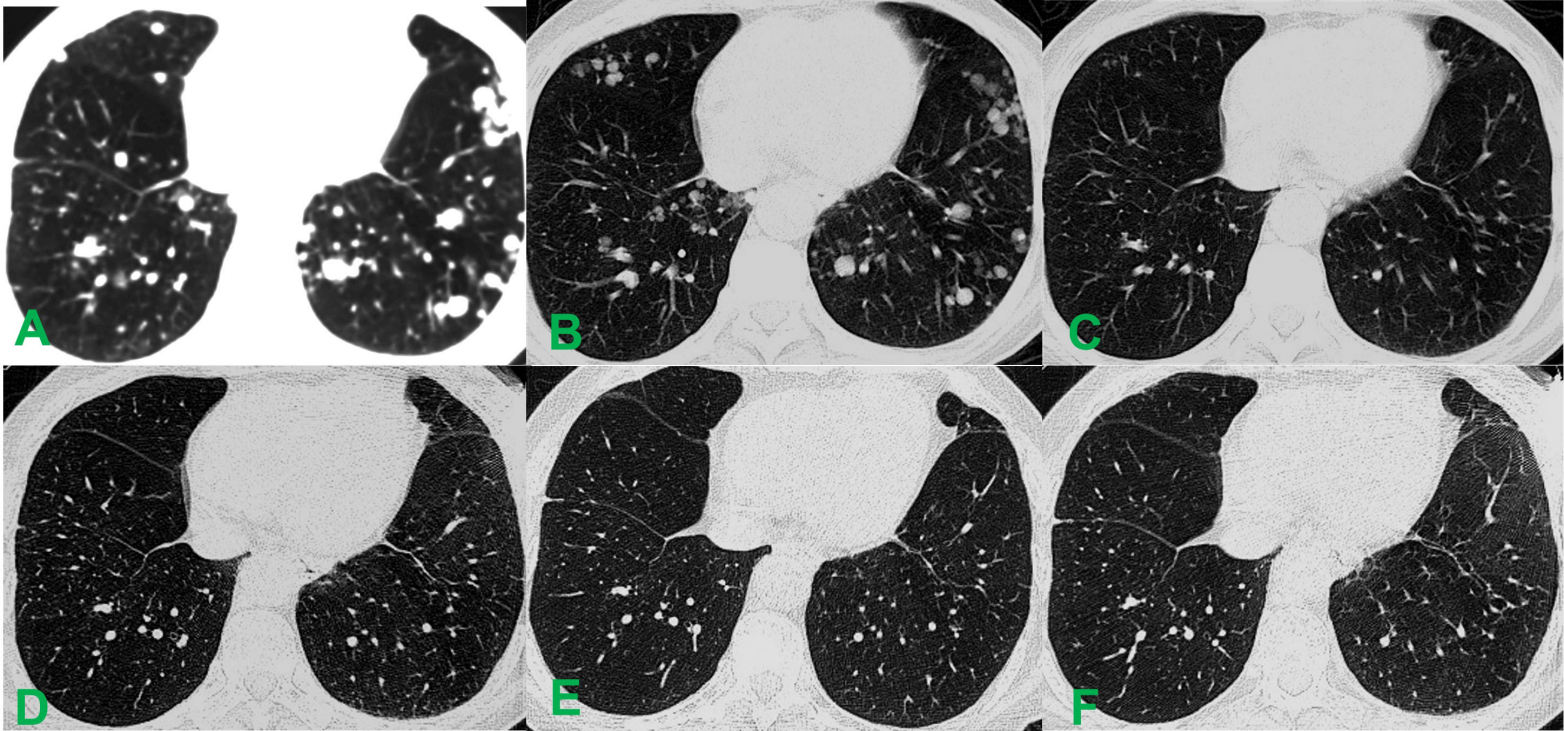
Comparative chest CT imaging before and after the intervention. (A) Pre-interventional pulmonary CT revealed numerous (>20) diffuse metastatic lesions in both lungs, with the largest measuring approximately 1.5 cm in diameter. (B) 4 weeks post-intervention, a reduction in both the number and volume of pulmonary metastatic lesions was observed, with the largest nodule measuring approximately 1.0 cm in diameter. (C) By 12 weeks after the procedure, the diffuse metastatic nodules had completely resolved. (D) No new lesions were detected on unenhanced CT at the 3-year follow-up. (E) Pulmonary CT imaging obtained 5 years post-intervention showed maintained remission. (F) At 10 years after treatment, follow-up CT demonstrated continued radiographic stability with no evidence of new or recurrent lesions.
